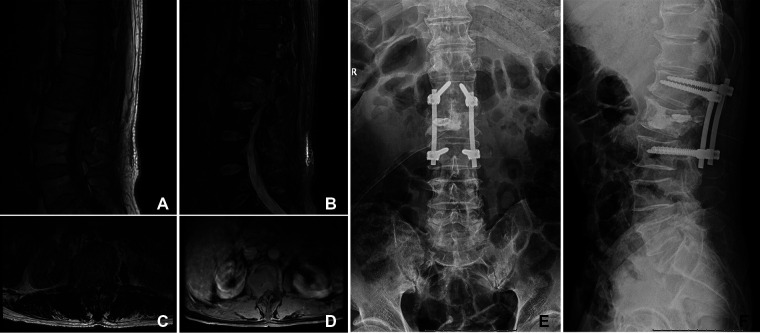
A 66-year-old woman suffered from HCC with multiple metastases of the spine and pelvis. The primary lesion of the liver was not resected. The symptoms were located in the lumbar 2 vertebra. She underwent orthopedic therapeutic surgery. (A–D) The L2 vertebral metastases in MRI. (E,F) The patient received intralesional resection with internal fixation and PKP. PKP, percutaneous kyphoplasty.
